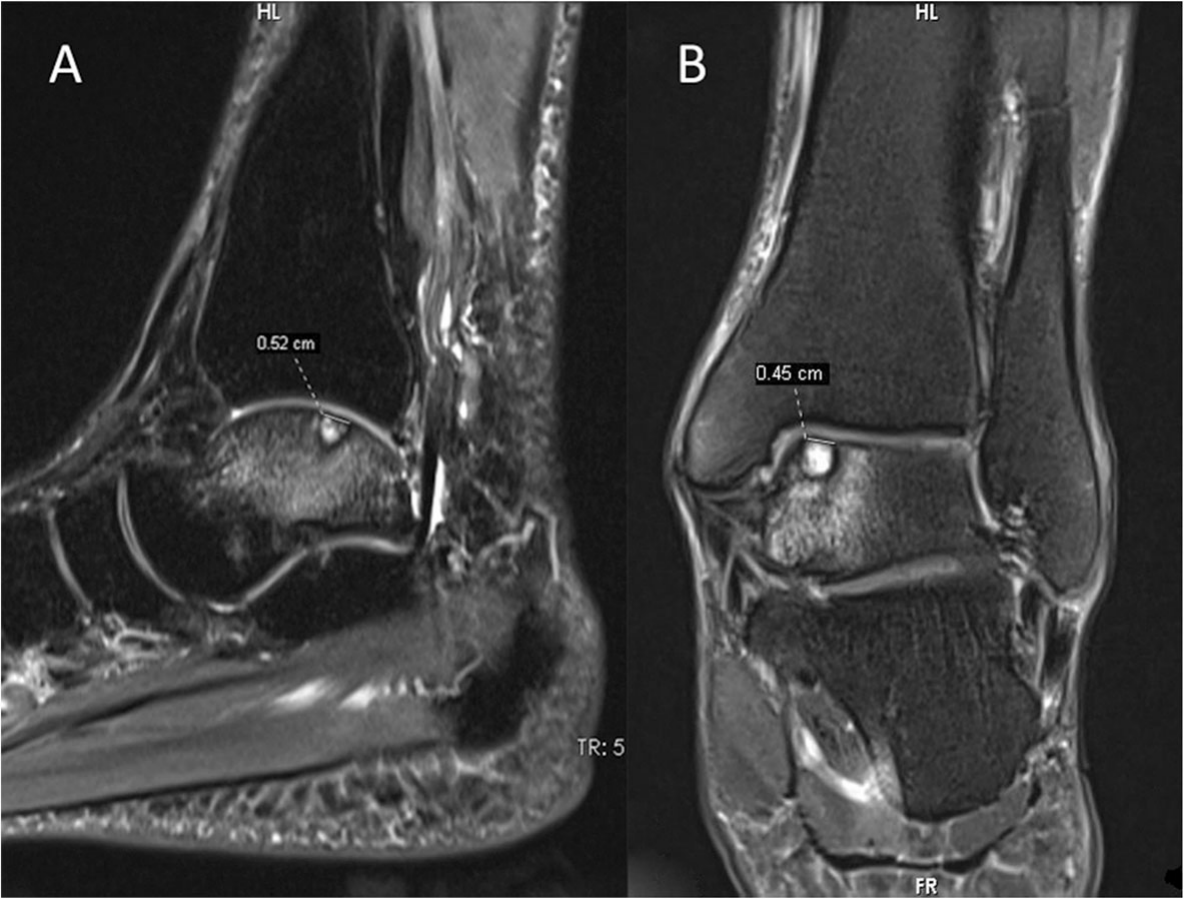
The diameter of OLT was measured on the sagittal (a) and coronal (b) imaging of MRI. The area of OLT was calculated by this equation: OLT area = coronal length × sagittal length × 0.79
